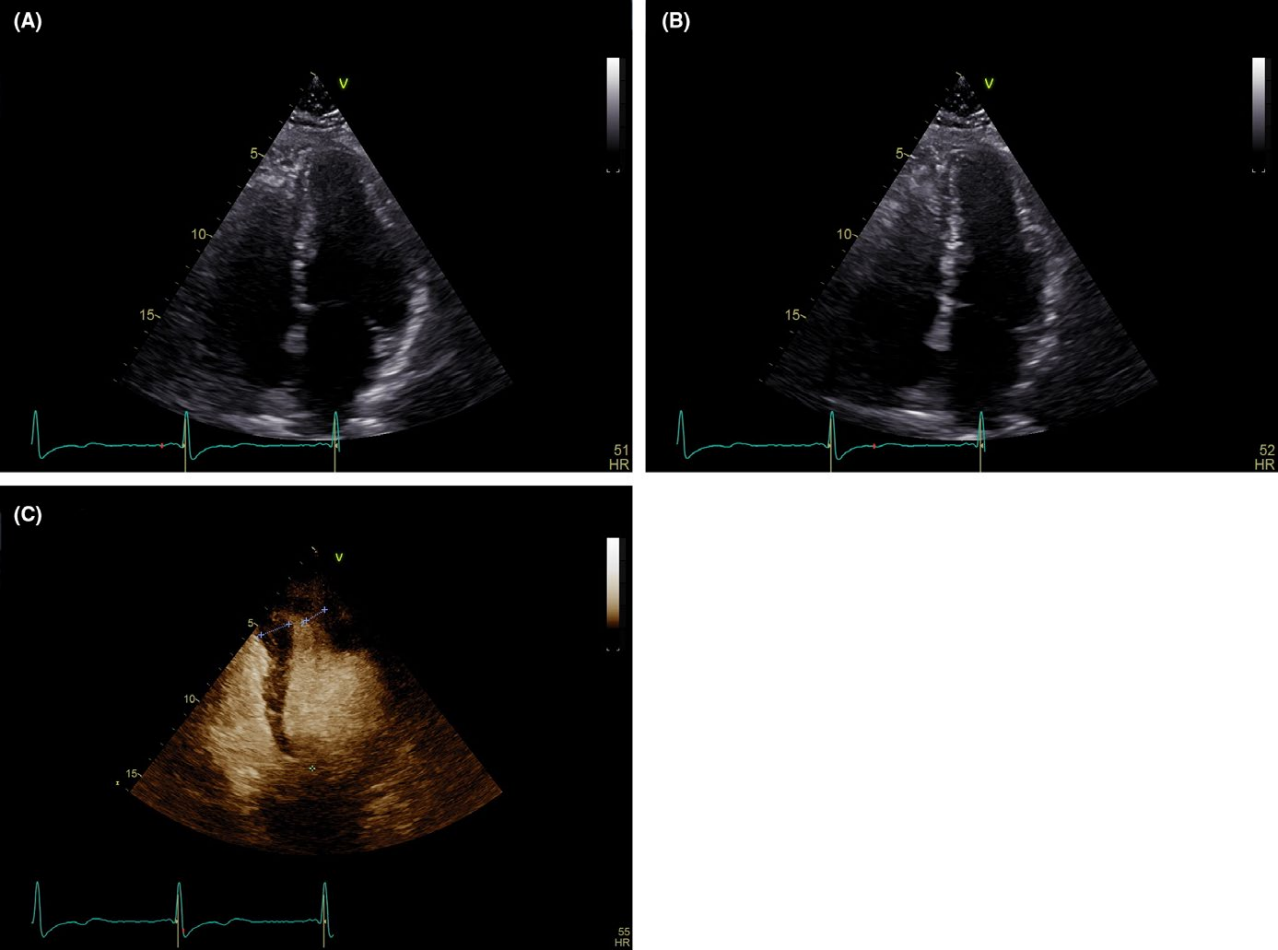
Transthoracic echocardiography in end‐diastole and end‐systole in the apical 4‐chamber view (A and B) Echocardiography with microbubble contrast revealing apical left ventricular hypertrophy (C)
